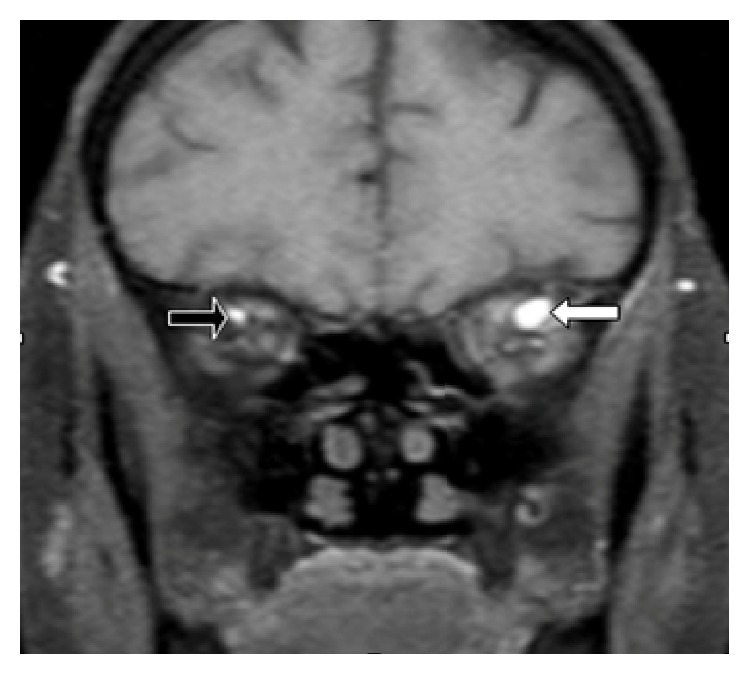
Axial T1-weighed magnetic resonance imaging with contrast demonstrating a greatly dilated left superior ophthalmic vein (white arrow) and normal right superior ophthalmic vein (black arrow).
